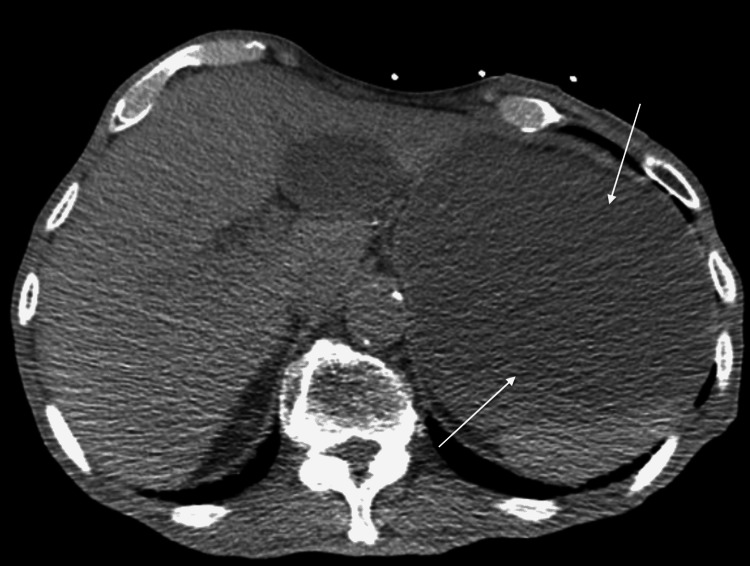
Dilated stomach "arrows" on admission computed tomography
